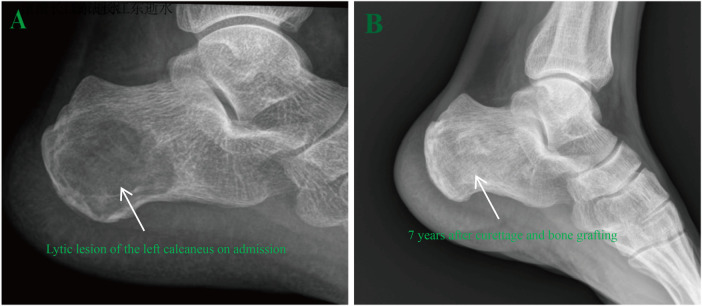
(A) A cystic lucency is noted within the posterior aspect of the left calcaneus, surrounded by a sclerotic border on x-ray. The adjacent soft tissue planes are preserved. (B) The bone cortex remains continuous, and the postoperative appearance after curettage and bone grafting shows no evidence of recurrence on x-ray at the 7-year follow-up. (Source: Original images obtained from the Department of Radiology).
